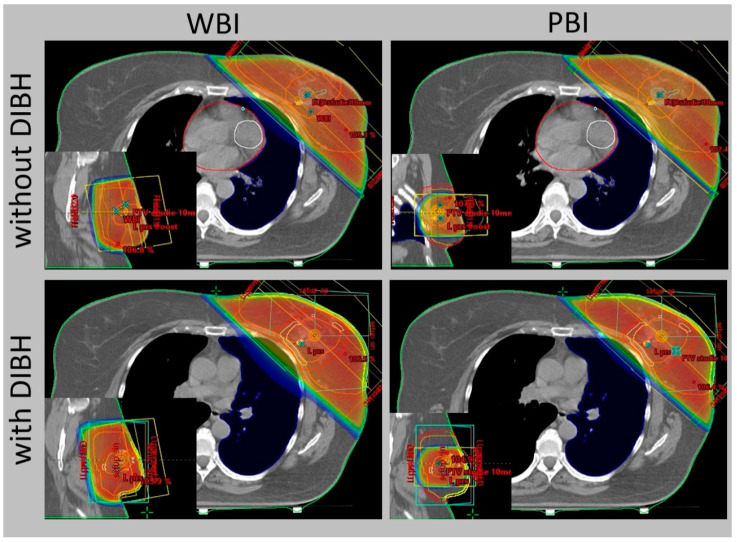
External-beam whole breast irradiation (WBI) and partial breast irradiation (PBI) with and without deep inspiration breath hold (DIBH)—comparison of the same target volume CT slice.
